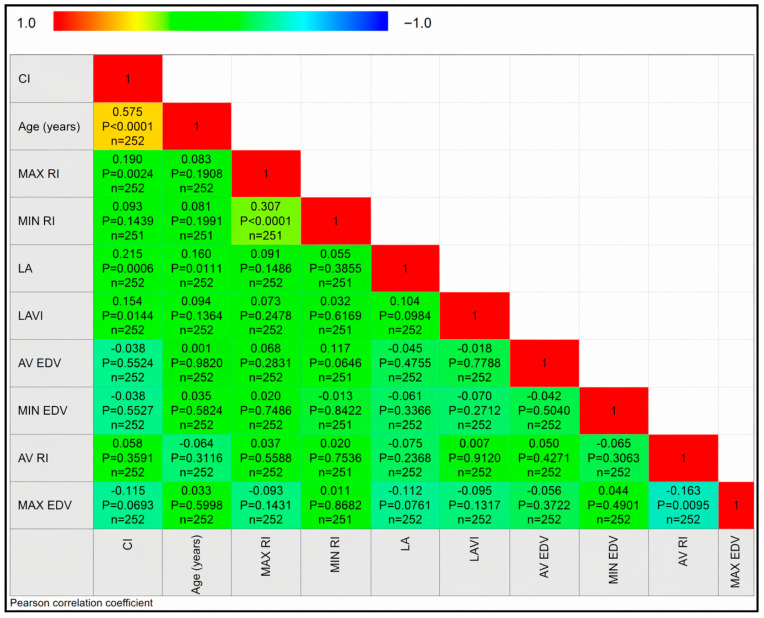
Correlation matrix displaying Spearman correlation coefficients between cognitive impairment (CI), age, echocardiographic indices, and transcranial Doppler parameters in atrial fibrillation patients. Colors represent the strength and direction of correlations.
